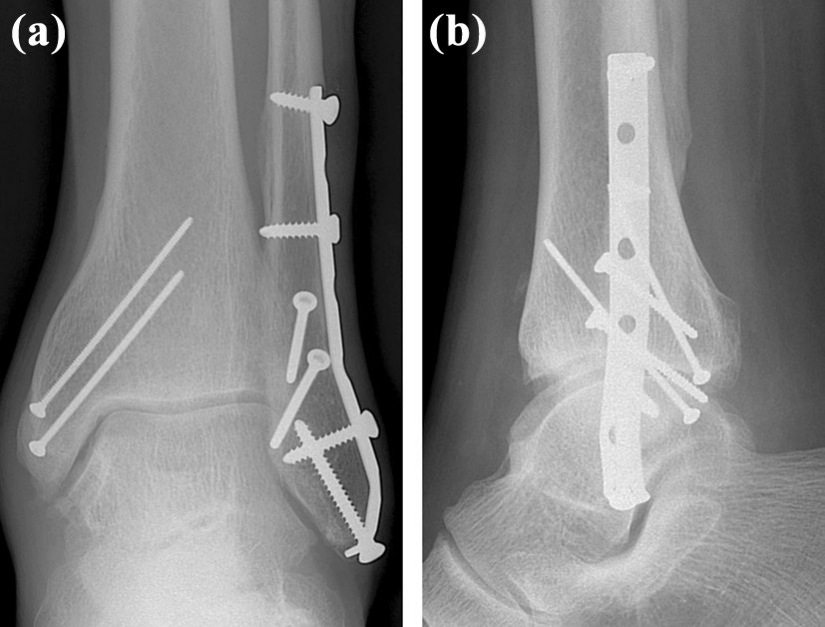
Postoperative radiographs after open reduction and internal fixation
